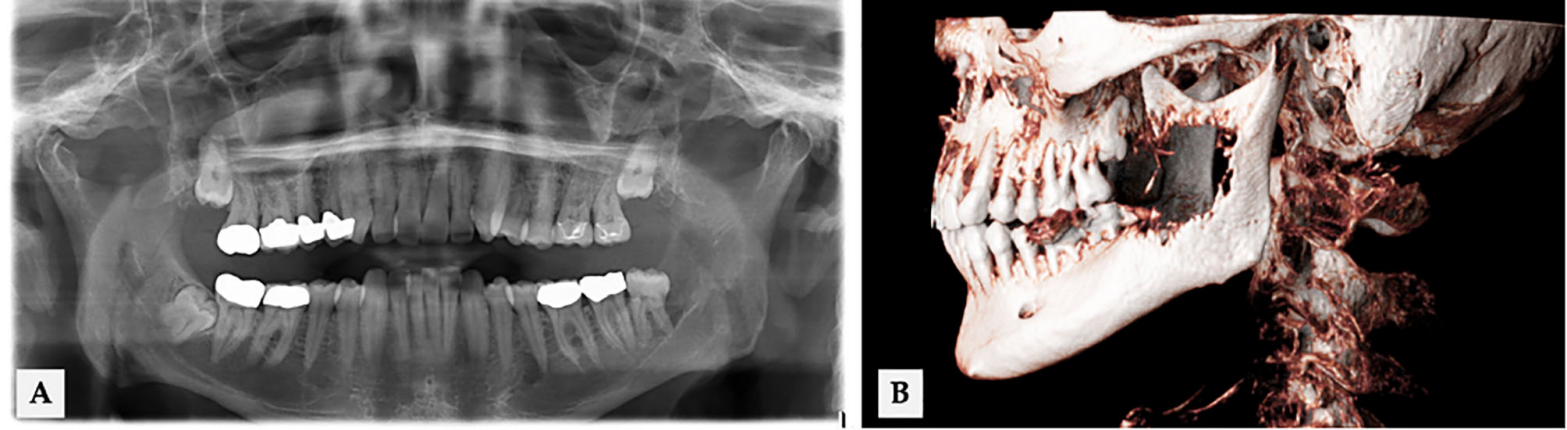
56 years old male with an infiltrative growth of oral squamous cell carcinoma (T4) in regio 38 (ID 18 in Figure 5). (A) Extension of osseous destruction in OPT and (B) cinematic volume rendering CT reconstruction.
